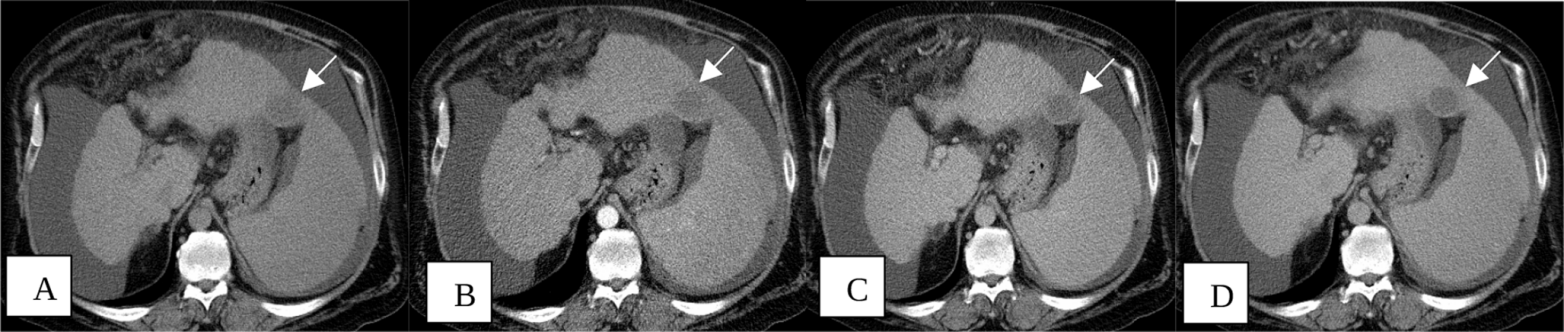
56-year-old male with cirrhosis, status post TACE for a 3.6 cm LI RADS 5 observation in segment III. Axial CT images before (A) and after intravenous contrast in the arterial (B), portal venous (B) and 3 min delayed (C) phases demonstrating a non-enhancing treatment cavity (white arrows) in keeping with LR-TR non-viable
